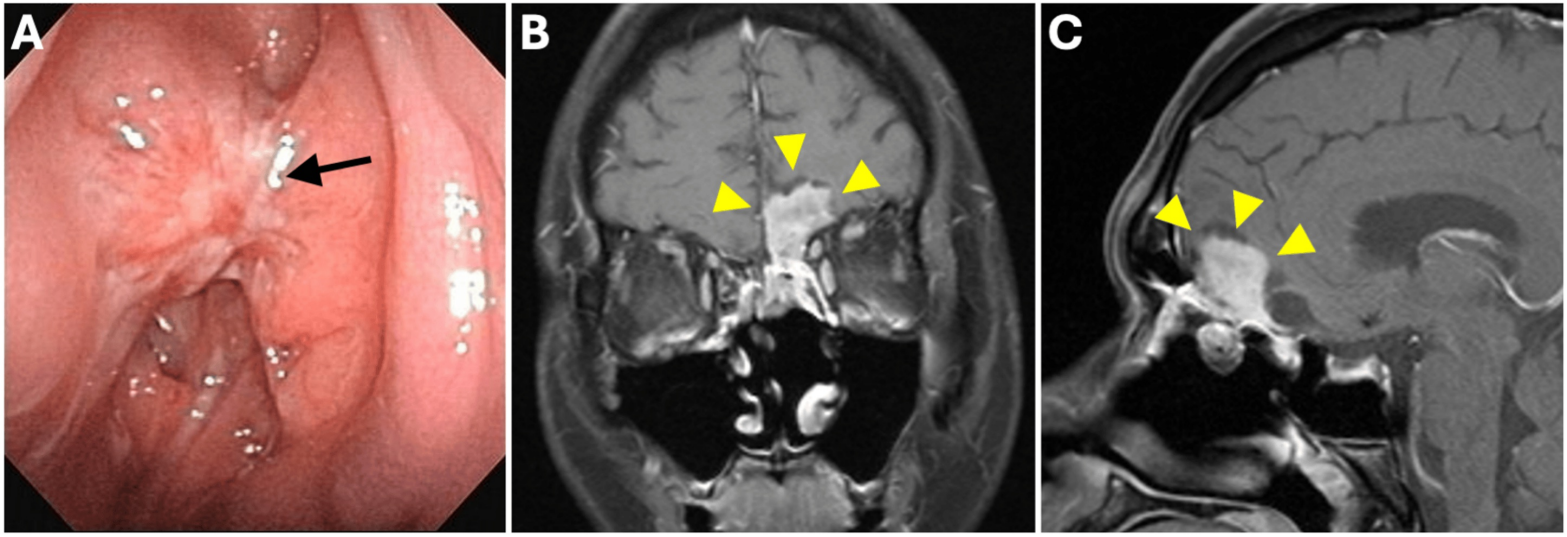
Findings after five cycles of neoadjuvant chemotherapy. (A) Nasal endoscopy confirmed a reduction in the size of the nasal cavity mass (black arrow). (B, C) Gadolinium-enhanced T1-weighted magnetic resonance imaging revealed marked reduction in the size of the lesions located in the nasal cavity, paranasal sinuses, and intracranial compartment, now measuring approximately 3.2 cm × 1.2 cm × 2.5 cm, with a persistent dumbbell configuration (yellow arrowhead). The lesion components within the left maxillary and frontal sinuses have nearly resolved, while those in the left ethmoid sinus and inferior aspect of the left frontal lobe show significant regression. The midline structures of the frontal lobe have reverted to their normal anatomical position. Post-contrast imaging demonstrates heterogeneous enhancement within the residual lesion.
